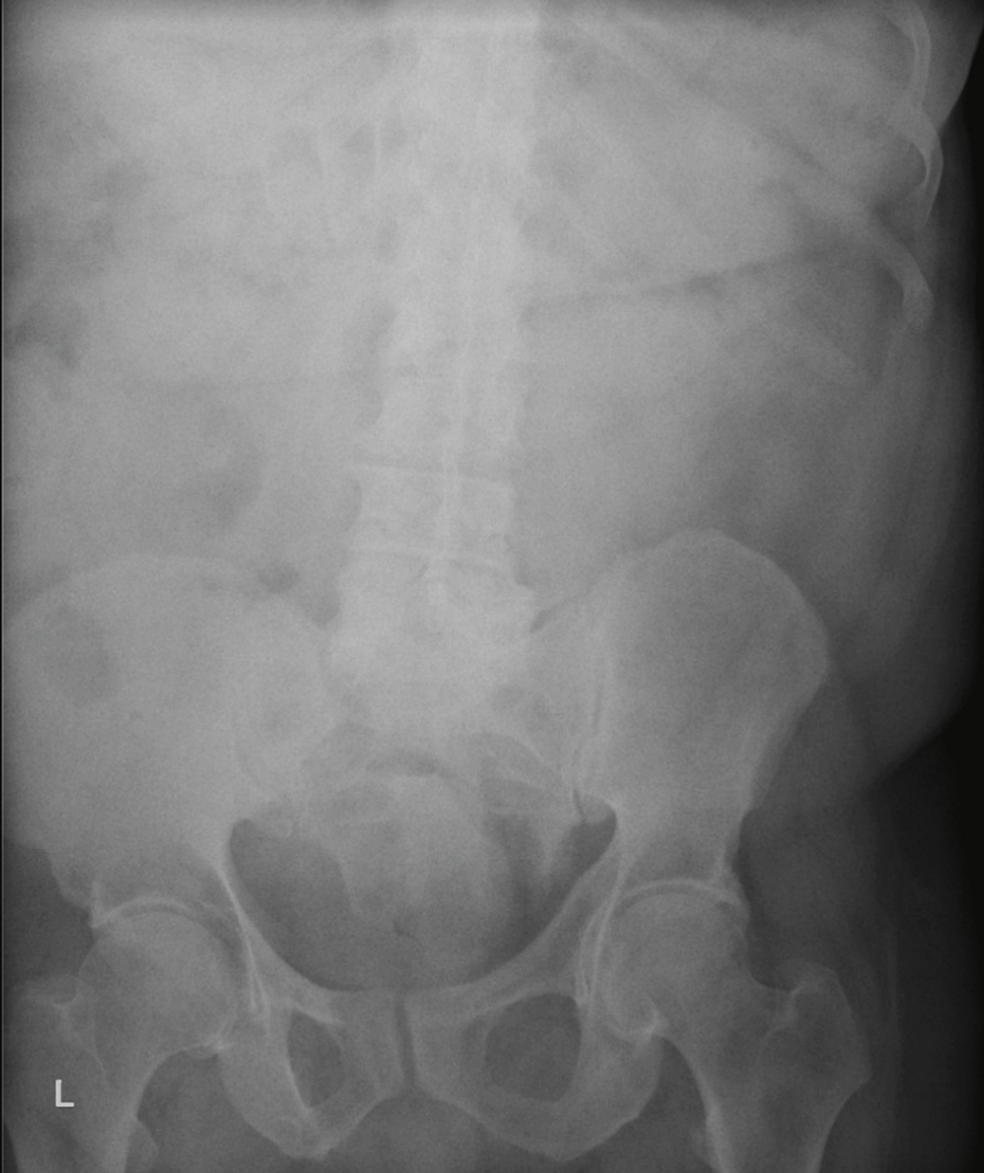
Radiograph after the passage of all batteries
